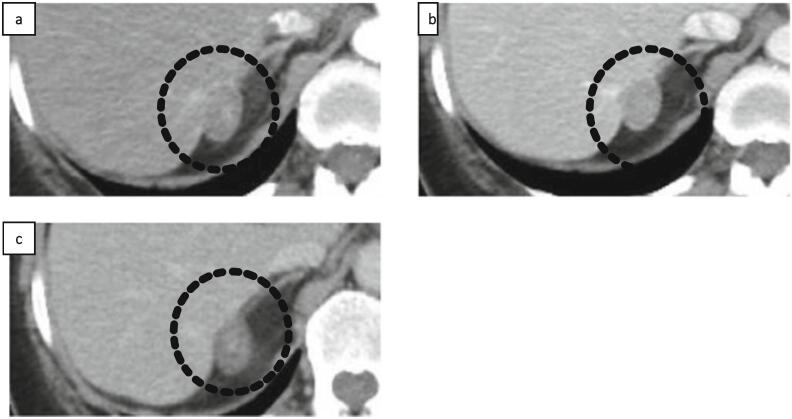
(a) Enhanced computed tomography (CT) revealed a 27-mm well-defined tumor located at liver segment 7, which was enhanced in the arterial phase. The enhancement was washed out in the (b) portal and (c) delayed phase (indicated by black dotted circle).
